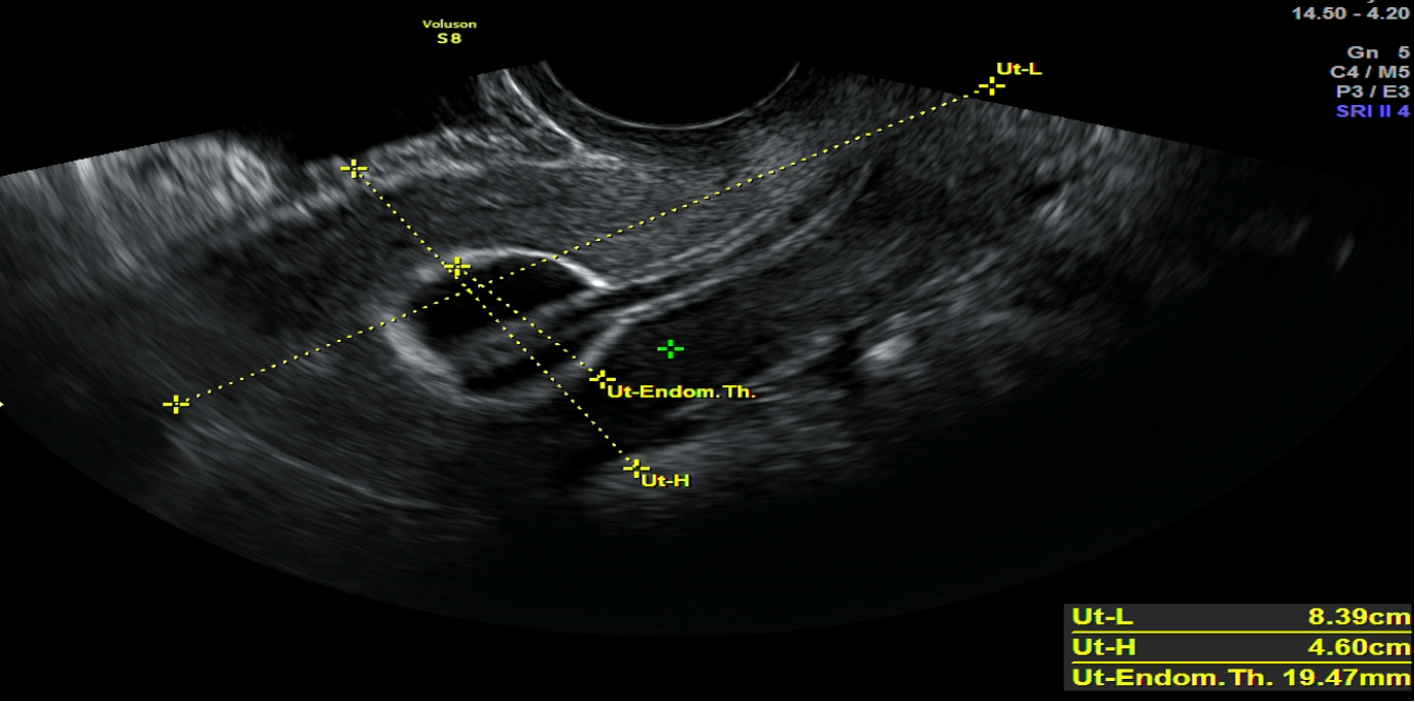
2D ultrasound image. A foley catheter balloon filled with 6 ml of normal saline in the reconstructed uterine cavity.
